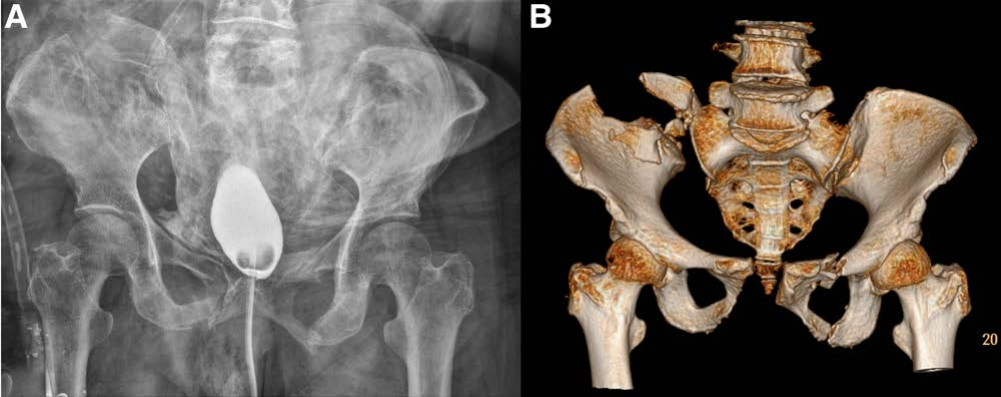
Initial radiographs. (A) Pelvis AP x-ray and (B) 3D reconstruction of computed tomography, revealing unstable pelvic ring injury. AP = anteroposterior.
